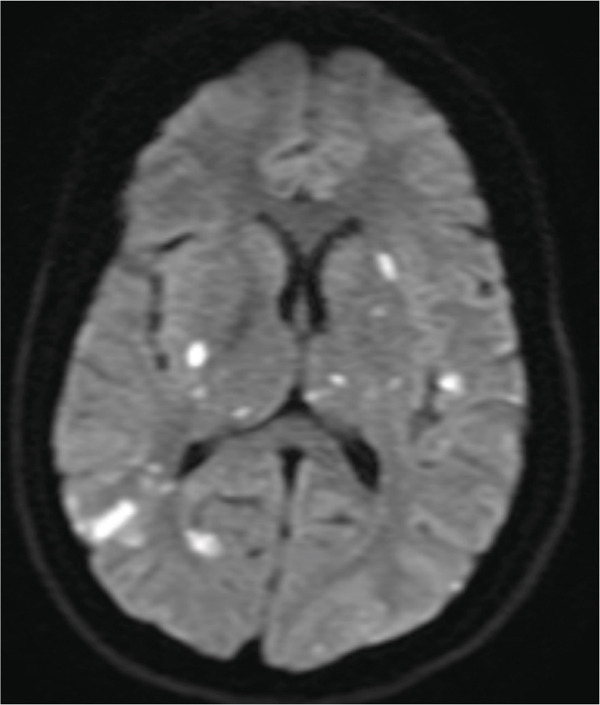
MRI axial cross section without contrast demonstrating diffuse infarcts.
